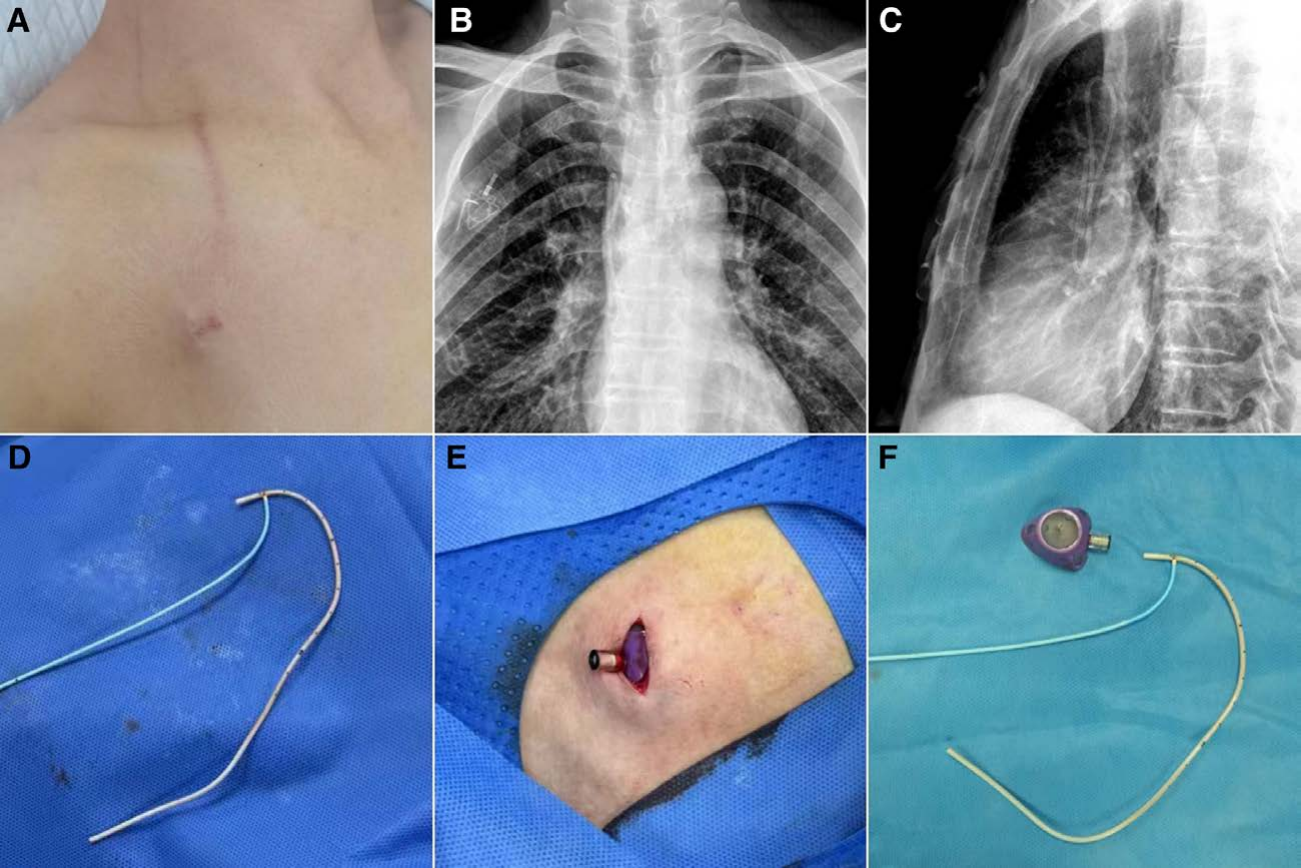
(A) The subcutaneous tunnel in front of the collarbone collapsed. (B) The frontal chest radiograph indicated that the catheter and the port body were disconnected. (C) The lateral chest radiograph showed that the broken catheter was approximately located in the SVC. (D) The catheter was removed by the snare device. (E) The isolated port body with a remaining part of the catheter; (F) The broken catheter and the isolated port body. SVC = superior vena cava.
